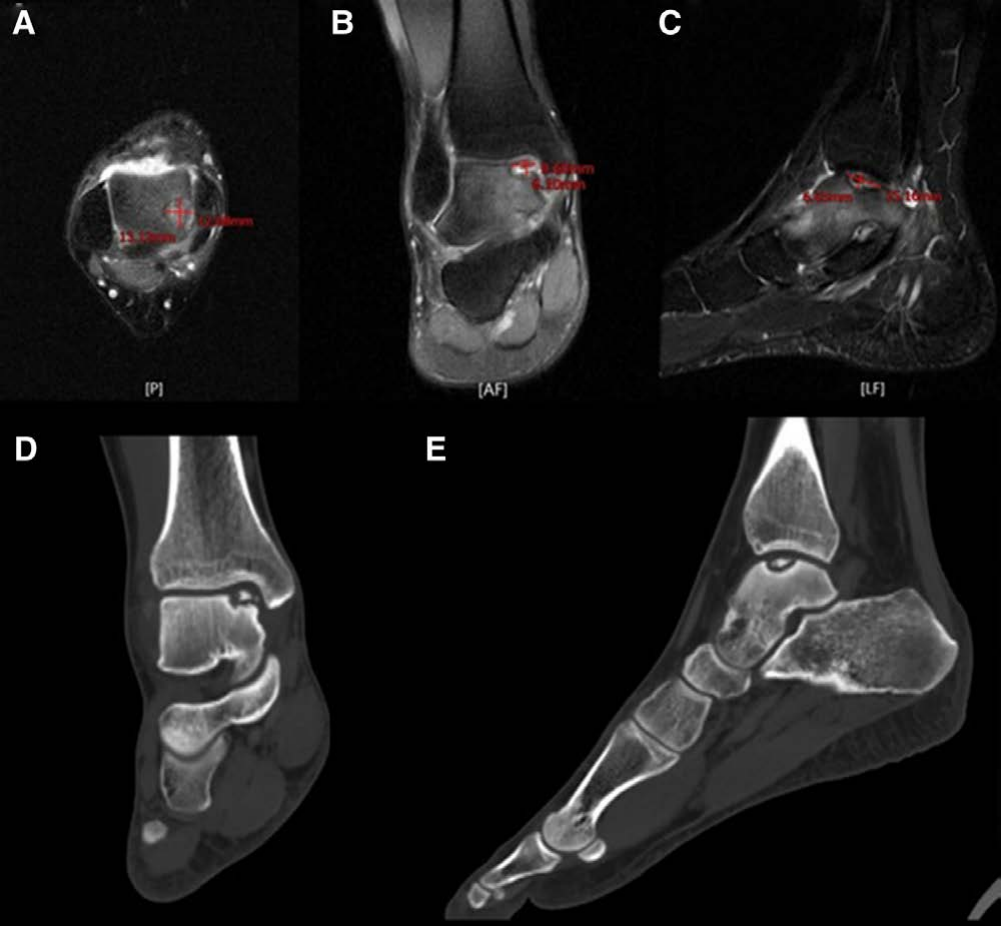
MRI, indicating a medial OCLT involving the dome of the talus (A–C); computed tomography of right ankle demo demonstrating the size and area of talus (D, E).
